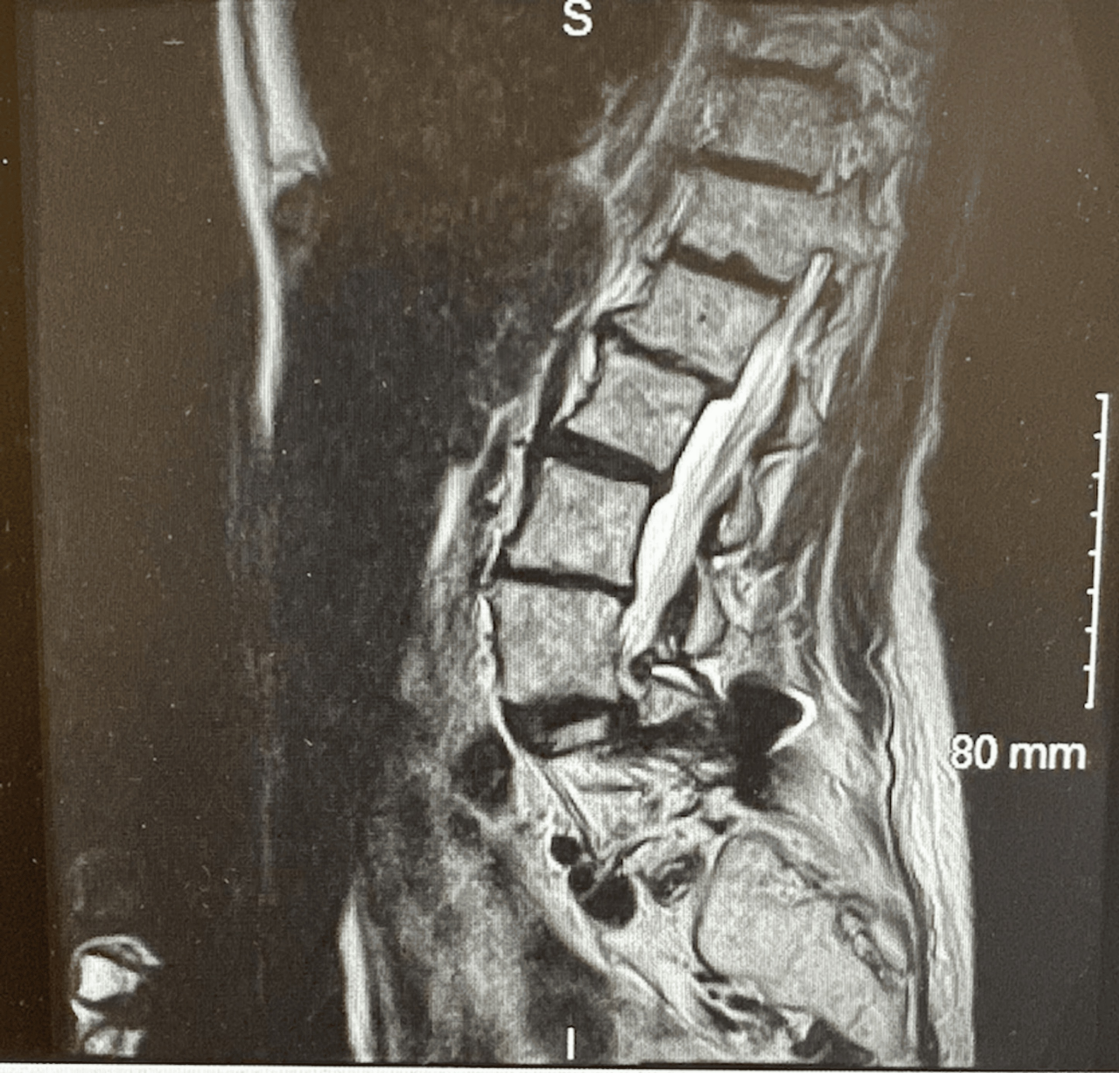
MRI of the lumbar spine MRI: magnetic resonance imaging Demonstrating multilevel severe degenerative changes in the lumbar spine
